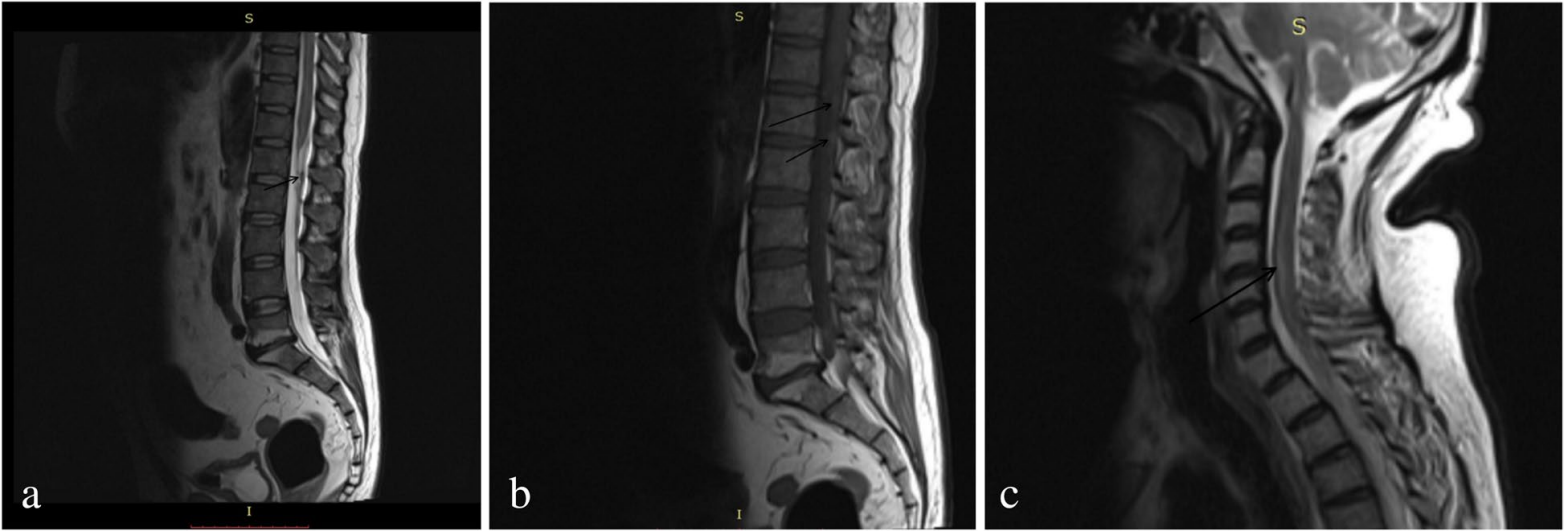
T2-weighted (a) and post-contrast T1-weighted (b) sagittal MRI of the lumbosacral spine showing nodular leptomeningeal lesions at lumbar level (L1), signal hyperintensities in the endplates of L5 and S1 with disc bulge at L4–L5 and L5–S1 levels causing thecal sac indentation; T2-weighted MRI of the cervical spine (c) showing an intramedullary hyperintense longitudinal lesion involving the central cord from C2 to C7 vertebral level, suggestive of leptomeningeal carcinomatosis
